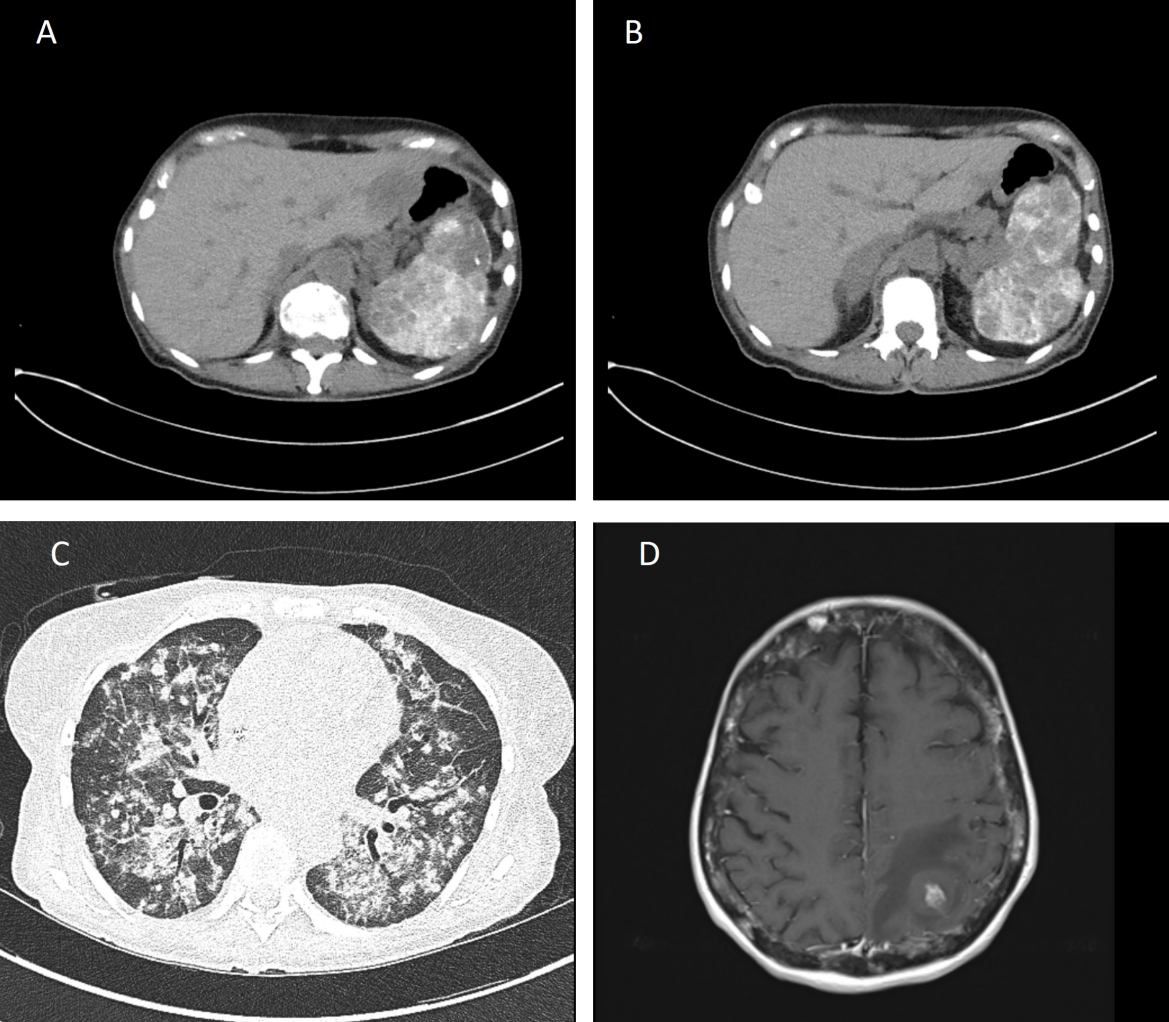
CT scans without contrast and MRI of the tumor lesions. (A, B) Upper left and right images of a CT scan of the abdomen and pelvis showing hypodense tumor lesions in the liver (A) and spleen (B). (C) Lower left image showing a chest CT scan of the lung with innumerable pulmonary nodules seen throughout the lungs. (D) MRI of the brain showing five supratentorial lesions with minimal enhancement within bilateral cerebral hemispheres. The largest lesion found within the superior left parietal lobe measured 2.4 cm in maximal dimension. Findings consistent with multiple intracranial hemorrhagic metastases with surrounding vasogenic edema.
